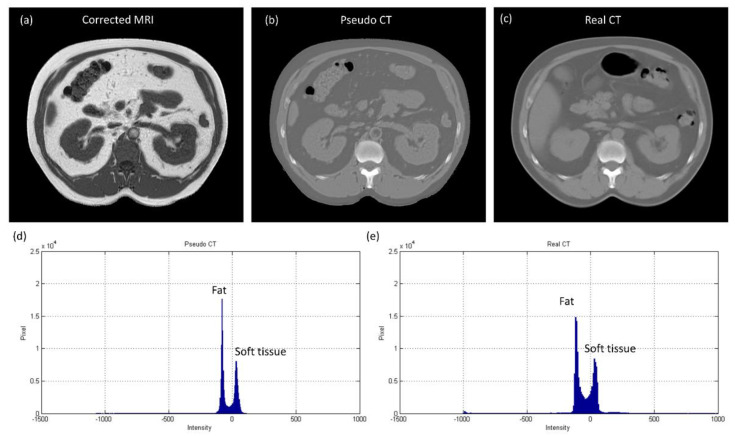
(a) Corrected MRI from 1.5 T scanner (WL/WW = 125/250), (b) pseudo-CT (WL/WW = 0/1000), (c) real CT (WL/WW = 0/1000), (d) histogram of pseudo-CT, and (e) histogram of real CT for abdominal imaging belonging to CNN testing dataset (${\mathrm{IMG}}_{\mathrm{testing}}^{\mathrm{abdomen}}$).
